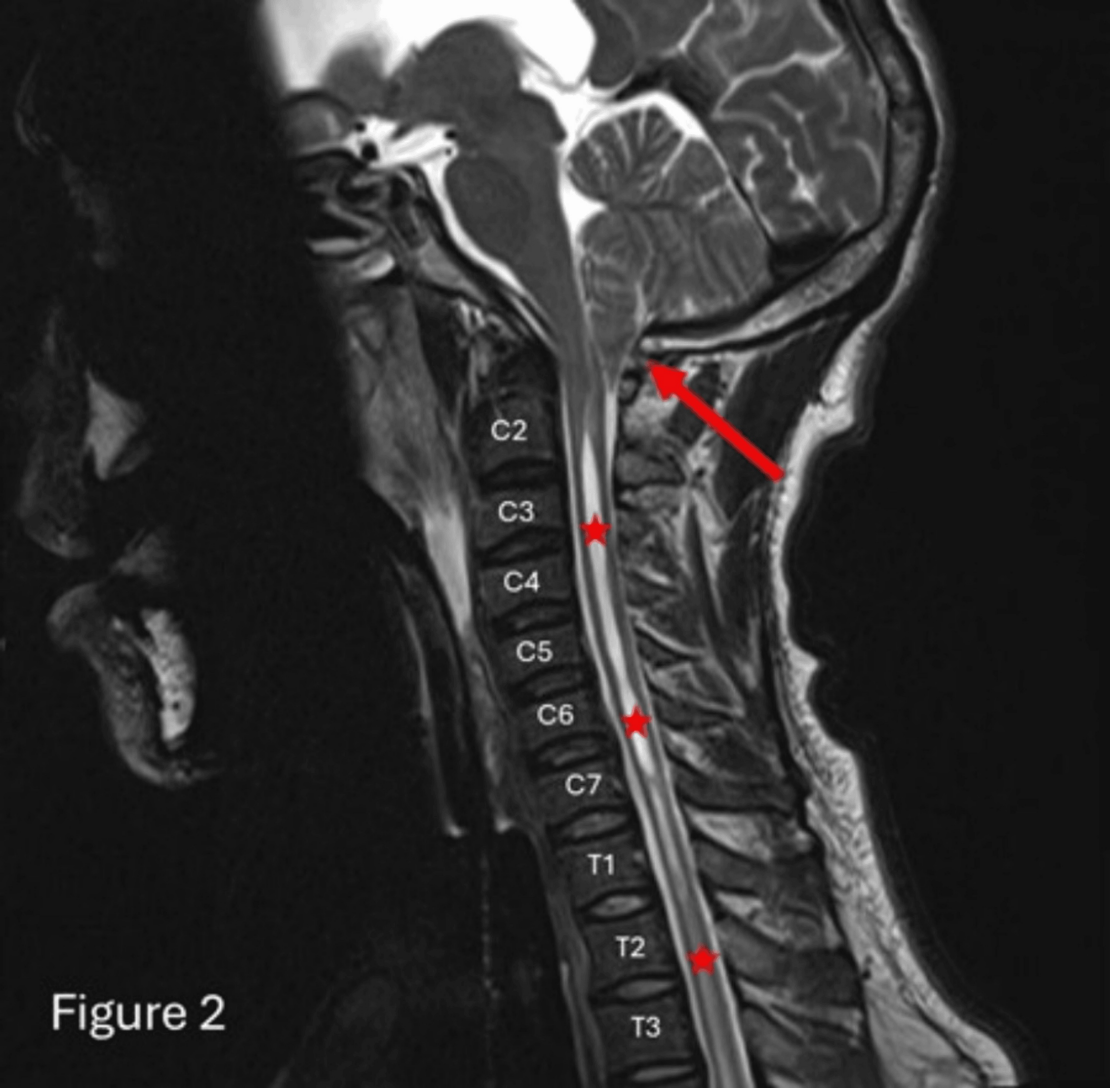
Sagittal T2-weighted MRI Sagittal T2-weighted MRI study of the cervical spine demonstrates a peg-like tonsil (red arrow) herniating across the foramen magnum with associated syrinx of the cervical and thoracic spinal cord (red asterisks) in keeping with Arnold-Chiari I malformation.
